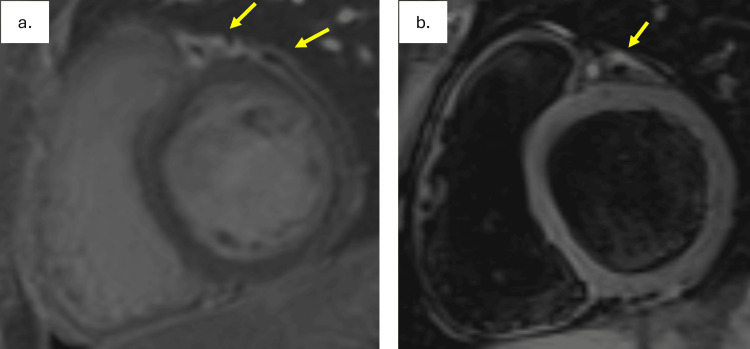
(a) No myocardial late gadolinium enhancement (LGE) was detected. There was LGE of the epicardial fat surrounding the proximal left anterior descending (LAD) artery with contiguous extension into the adjacent parietal pericardium (arrows), likely reflecting local inflammatory changes related to peri-coronary vasculitis. (b) T2-weighted imaging demonstrated evidence of inflammation and edema surrounding the proximal LAD (arrow).
